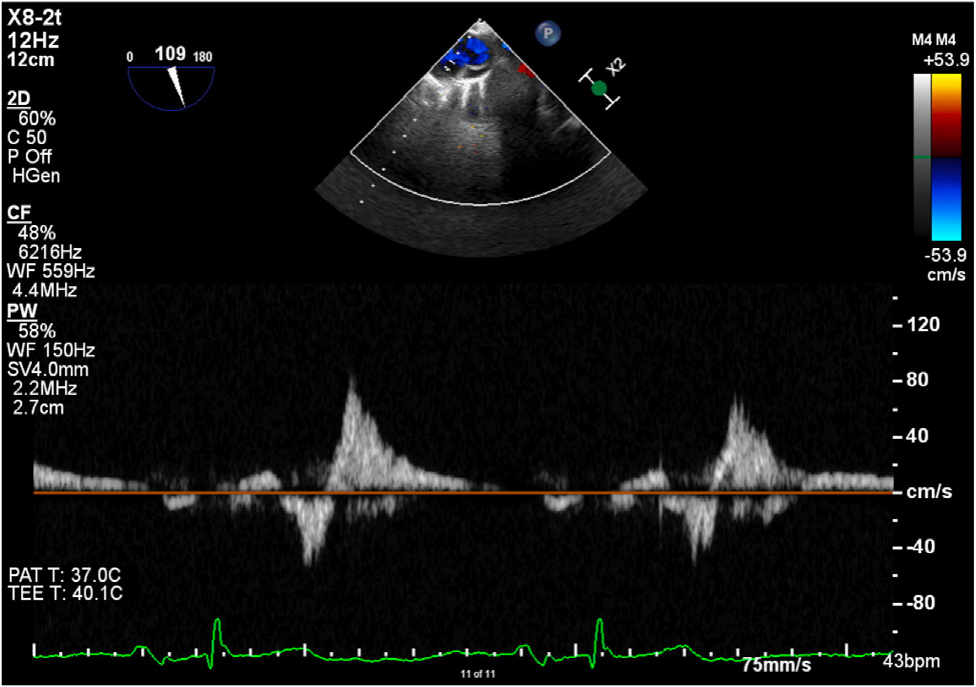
Two-dimensional TEE, midesophageal long-axis (109°) view of the pulmonary veins with color flow Doppler-guided pulsed-wave spectrum, demonstrates systolic flow reversal of the severe MR.
